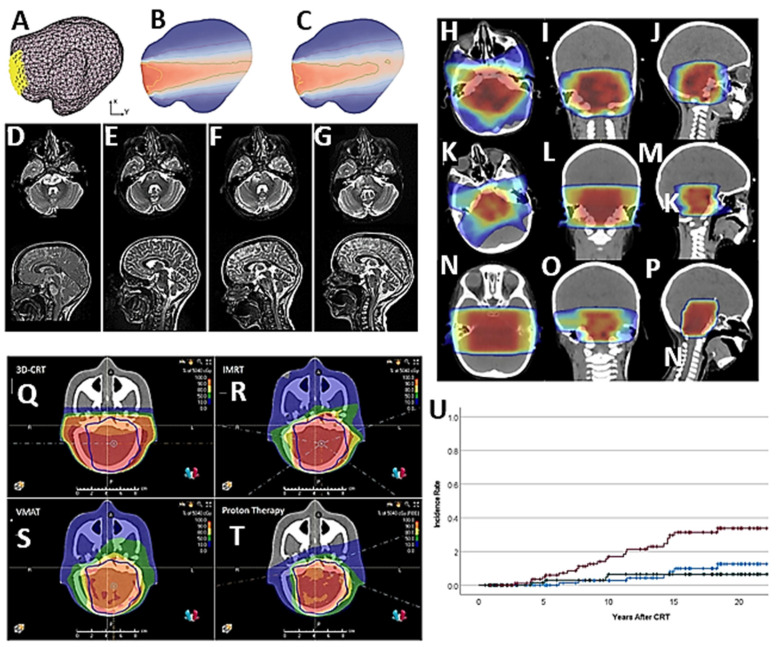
Clinical studies. Advances in RT for pediatric HGG patients. (A–C) Dose distribution in real geometries of DIPG tumors irradiated from a single patch. (A) Meshed geometry with an initial patch shown in yellow; (B) dose distribution considering homogeneous DIPG tumor properties; (C) dose distribution considering heterogeneous DIPG tumor properties (after [54], with permission). (D–G) Imaging of the tumor in T2w-MRI axial and sagittal at the following timepoints: (D) initial diagnosis 01/2020; (E) 6 months after the initial irradiation, (F) progression 01/2021; (G) 1 month after the second re-irradiation (after [55], with permission). (H–P) First irradiation isodose distribution in axial (H), coronal (I), and sagittal (J): 20 Gy (dark blue), 32 Gy (light blue), 40 Gy (green), 45 Gy (yellow), 48 Gy (orange), and 54 Gy (red). Second irradiation isodose distribution in axial (K), coronal (L), and sagittal (M): 7.5 Gy (dark blue), 12 Gy (light blue), 15 Gy (green), 17.5 Gy (yellow), 19 Gy (orange), and 22 Gy (red). Third irradiation isodose distribution in axial (N), coronal (O), and sagittal (P): 4 Gy (dark blue), 8 Gy (light blue), 9 Gy (green), 10 Gy (yellow), 11 Gy (orange), and 12 Gy (red) (after [56], with permission). 3D-CRT (Q), IMRT (R), VMAT (S), and PBS proton therapy (T) plans showing isodoses and PTV (blue) as computed by the treatment planning system (after [57], with permission). (U) Incidence rate for the development of any neoplasm (upper curve, red), meningioma (middle curve, blue), or HGG (lower, green) after conformal photon RT for ependymoma, age < 3 years (after [58], with permission).
